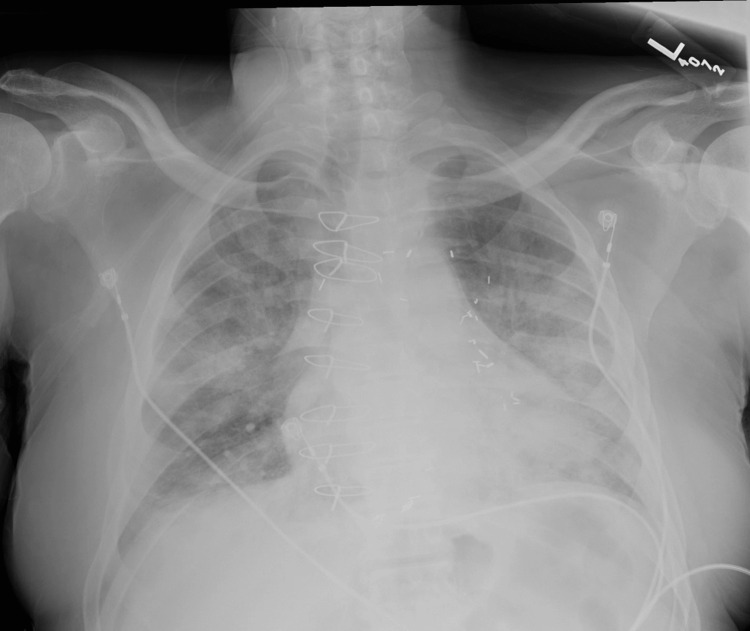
Patient's Chest X-ray on Initial Presentation Patient was determined to have bilateral pulmonary opacities most concerning in conjunction with his clinical presentation for acute respiratory distress syndrome or multifocal pneumonia.
